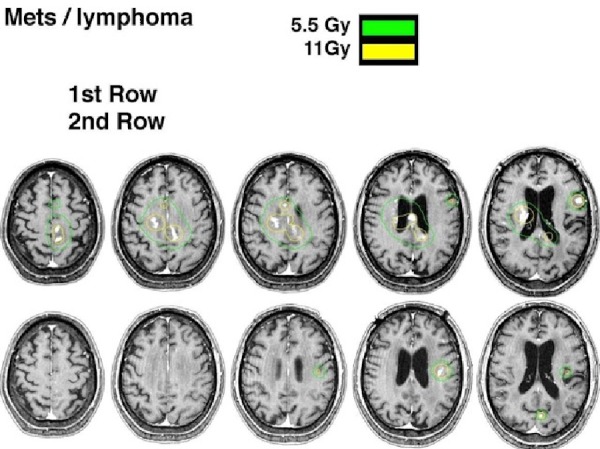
Brain MRI before and two months after GKRS treatment
